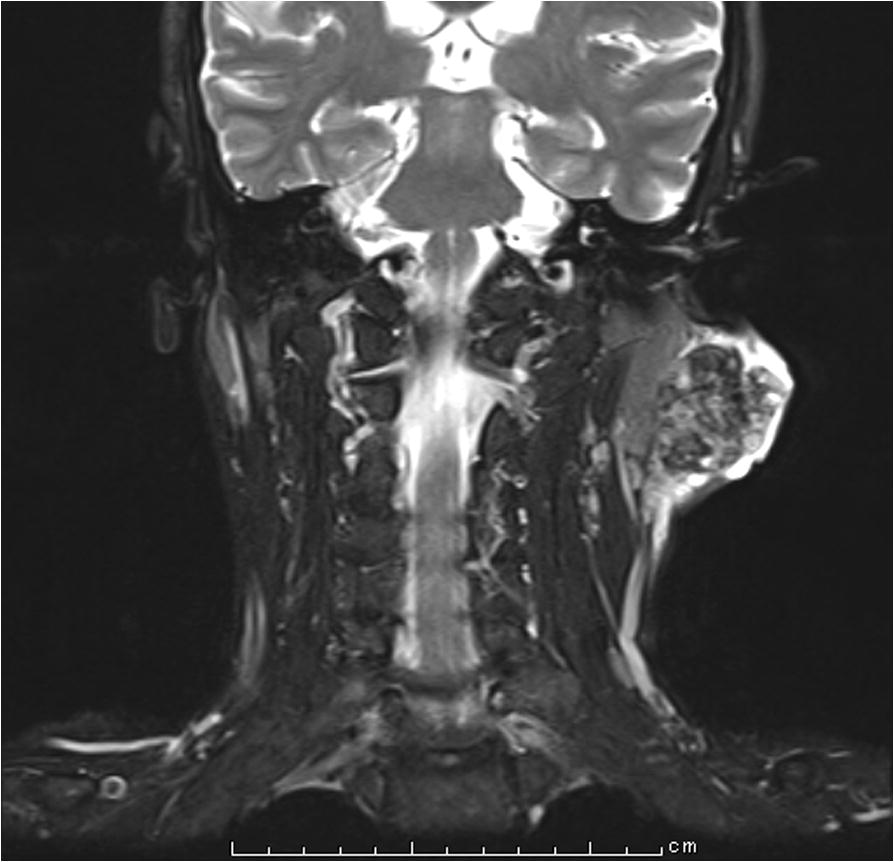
T1-weighted magnetic resonance imaging (MRI) of the left parotid region. A well-defined multilocular tumor with heterogeneous enhancement was seen. The tumor was located close to the outside of the left parotid gland
